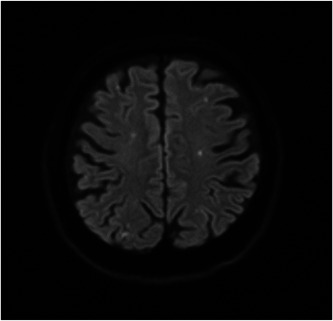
Magnetic resonance image of the head taken at on hospitalization day. There are multiple small acute brain infarctions distributed in both hemispheres indicating Trousseau syndrome.
